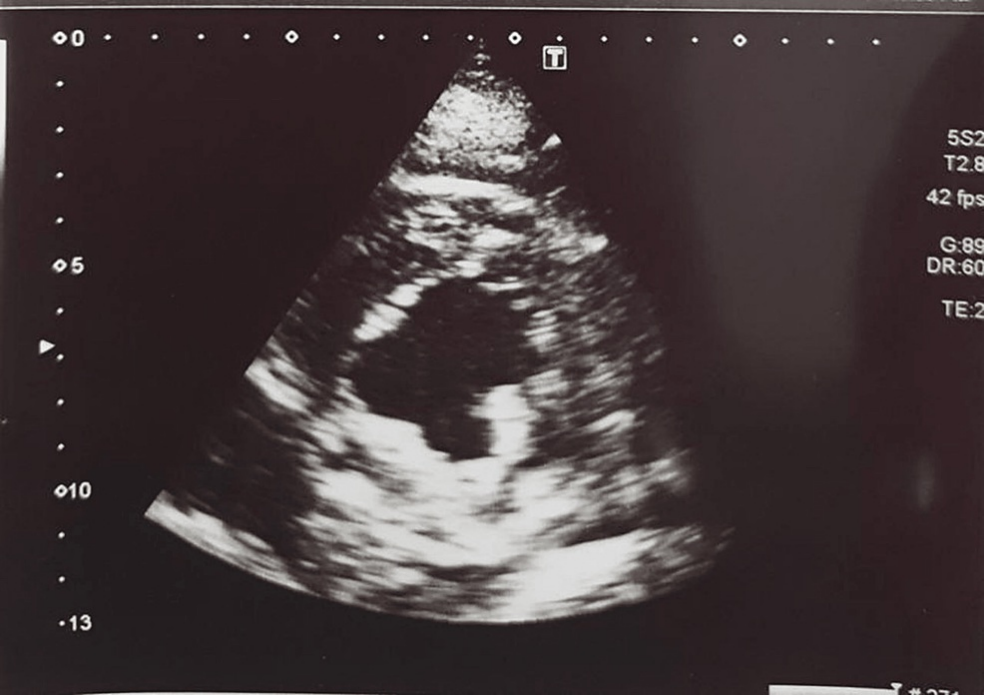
The two-dimensional echocardiogram showing mild left ventricular dysfunction.
